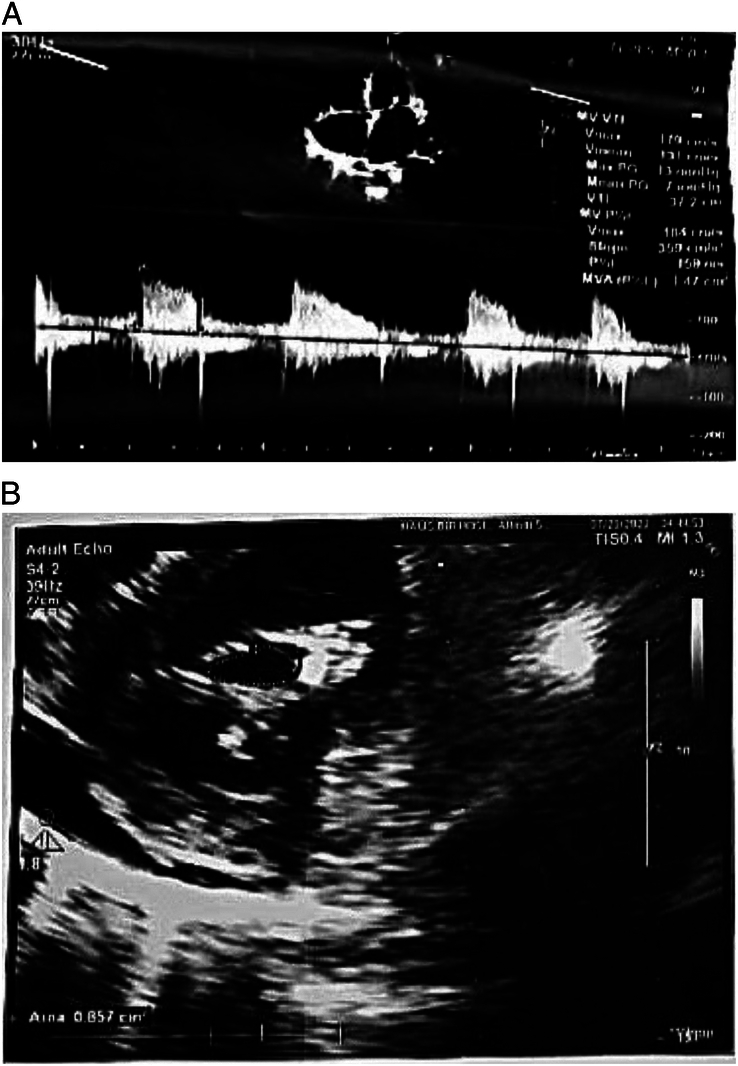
(A) Echocardiography findings with a 4-chamber view showing both mitral stenosis and atrial septal defect. (B) Echocardiography findings of parasternal short axis views showing stenosed mitral valve.
